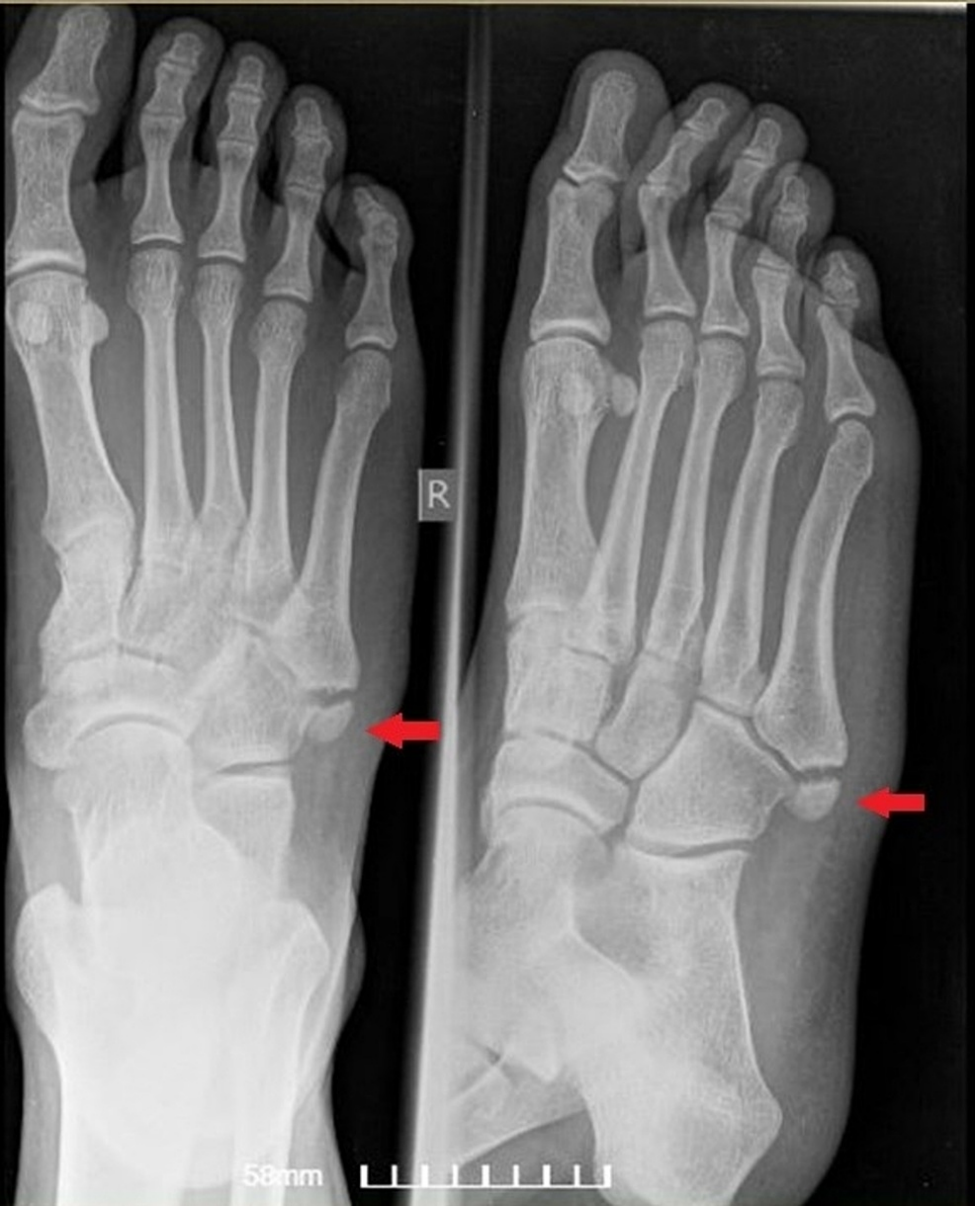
Plain radiograph (AP and lateral oblique) of the contralateral foot reveals the presence of a similar os vesalianum pedis (“mirror image”) (red arrow). AP: anteroposterior
